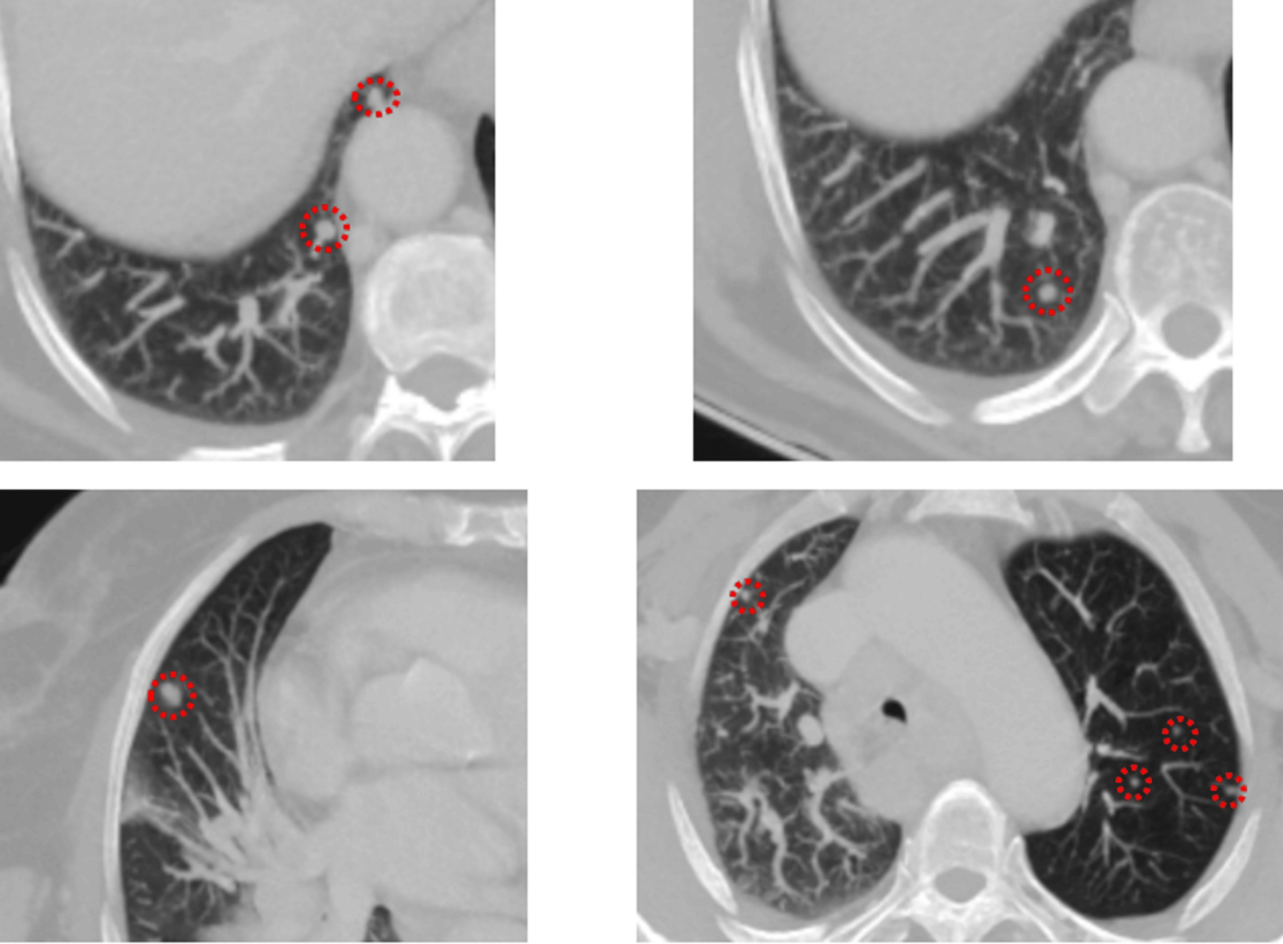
Axial and magnified CT images showing multiple bilateral pulmonary parenchymal nodules (circles), consistent with secondary metastatic lesions.
